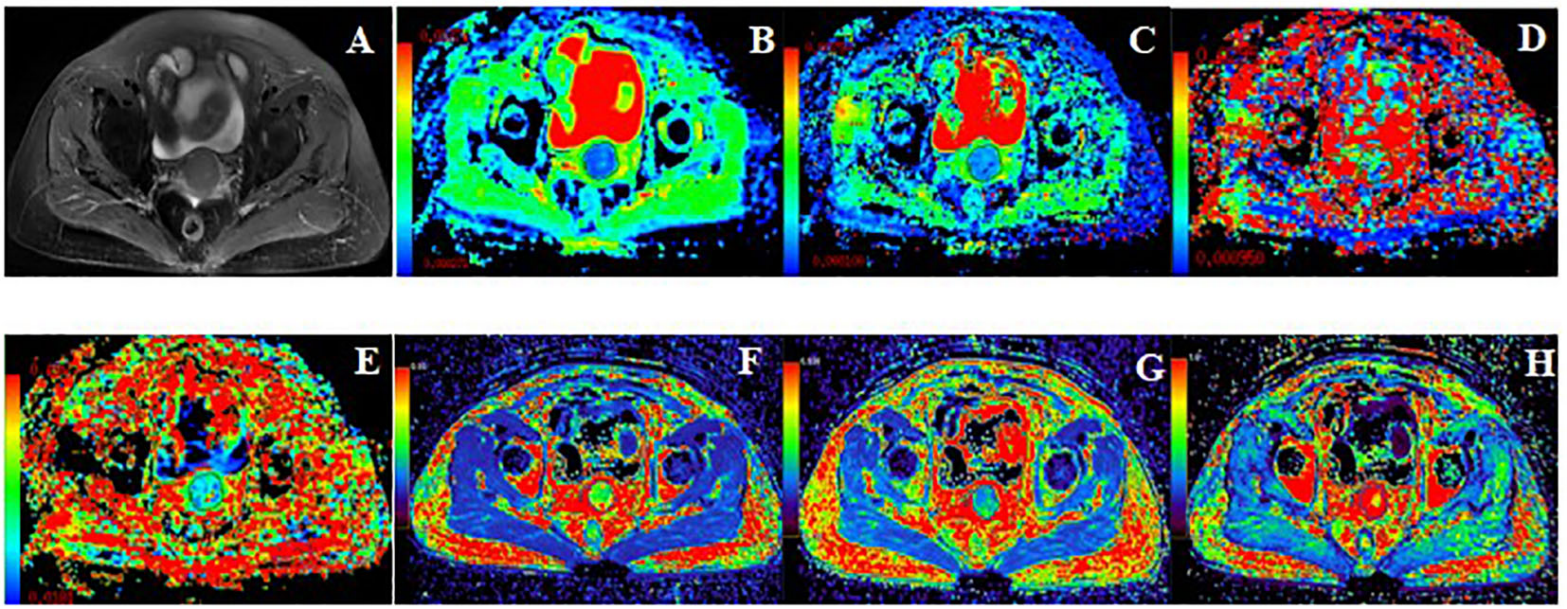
62 years old, FIGO IIA2 cervical cancer without PLNM. (A): Axial T2WI image. (B–H): Pseudo-color images of each quantitative parameter of DCE-MRI and IVIM-DWI, (A): Axial T2WI image, (B): ADC_min_ was 0.67×10^-3^mm^2^/s, ADC_mean_ was 0.77×10^-3^mm^2^/s, ADC_max_ was 1.10×10^-3^mm^2^/s (C): D was 0.61×10^-3^mm^2^/s, (D): D* was 30.00×10^-3^mm^2^/s, (E): f was 0.15, (F): K^trans^ was 1.19 min^-1^, (G): V_e_ was 0.35, (H): K_ep_ was 3.56 min^-1^.
